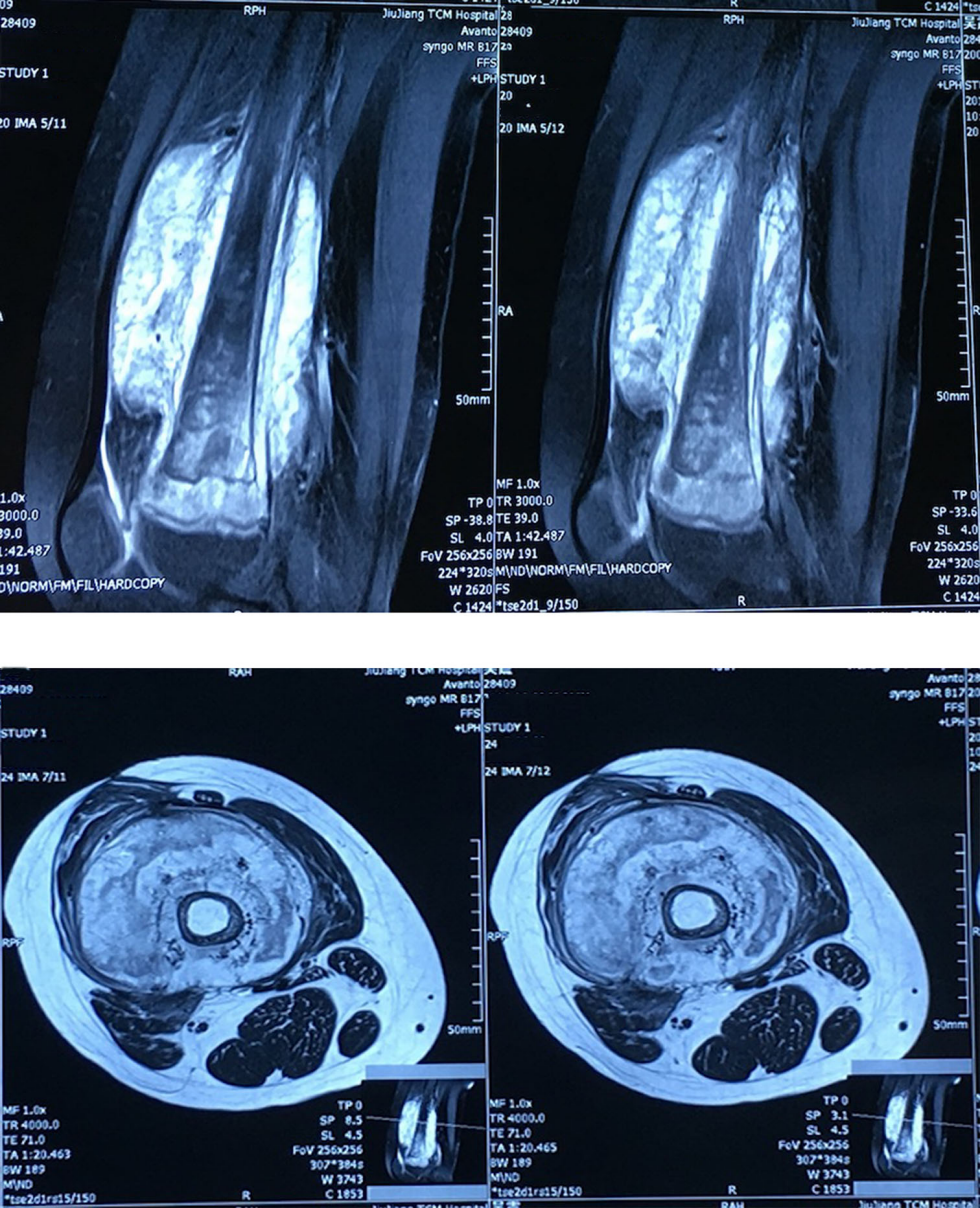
Enhanced magnetic resonance imaging (MRI) of the right thigh revealed bone thinning of the right femur, surrounding a soft tissue mass shadow around the femur and high possibility of malignant tumor.
